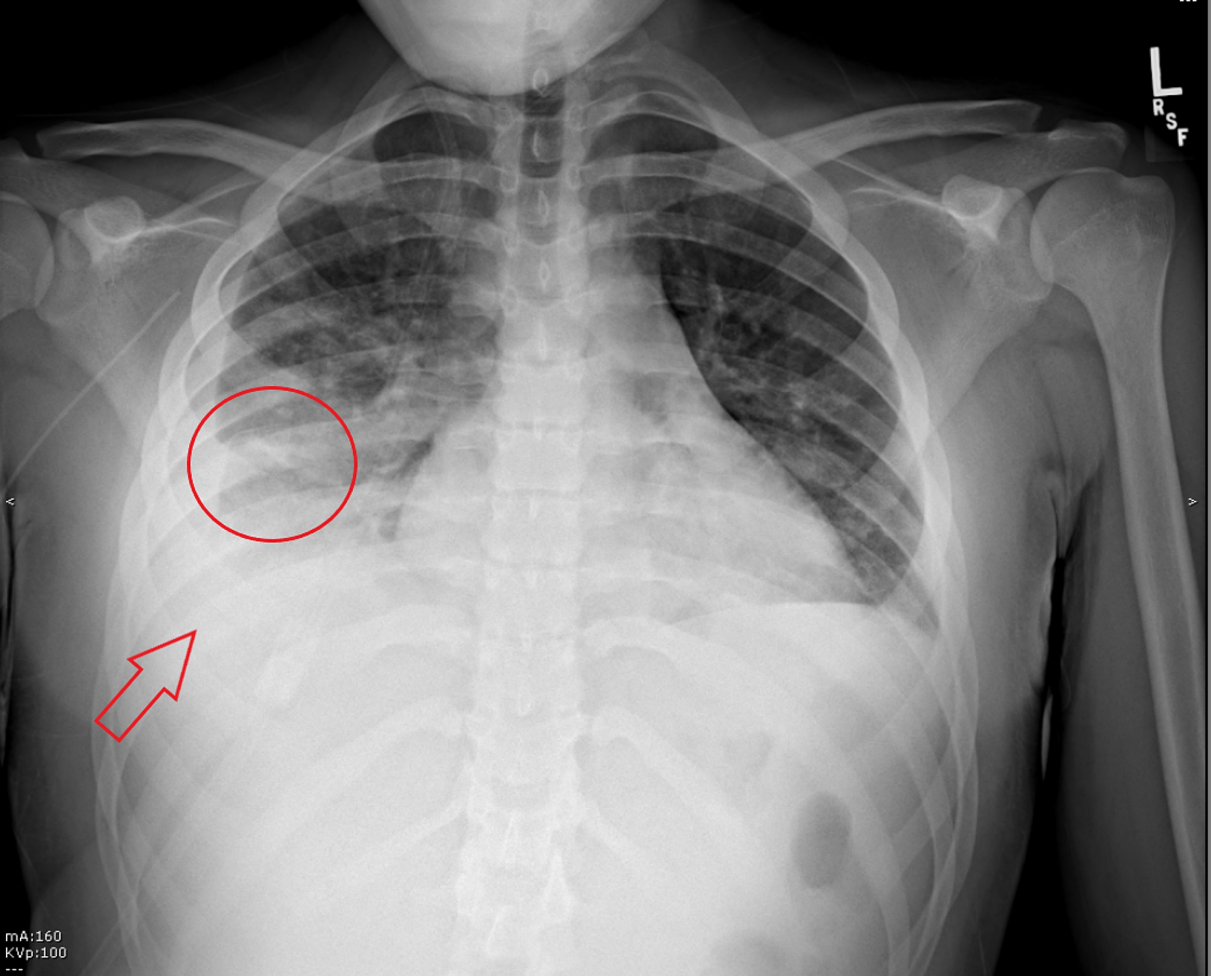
Frontal radiograph demonstrates patchy opacification (circle) with a relative central lucency (circle). Additionally, there is a small-moderate volume right pleural effusion (arrow) with adjacent compressive atelectasis.
